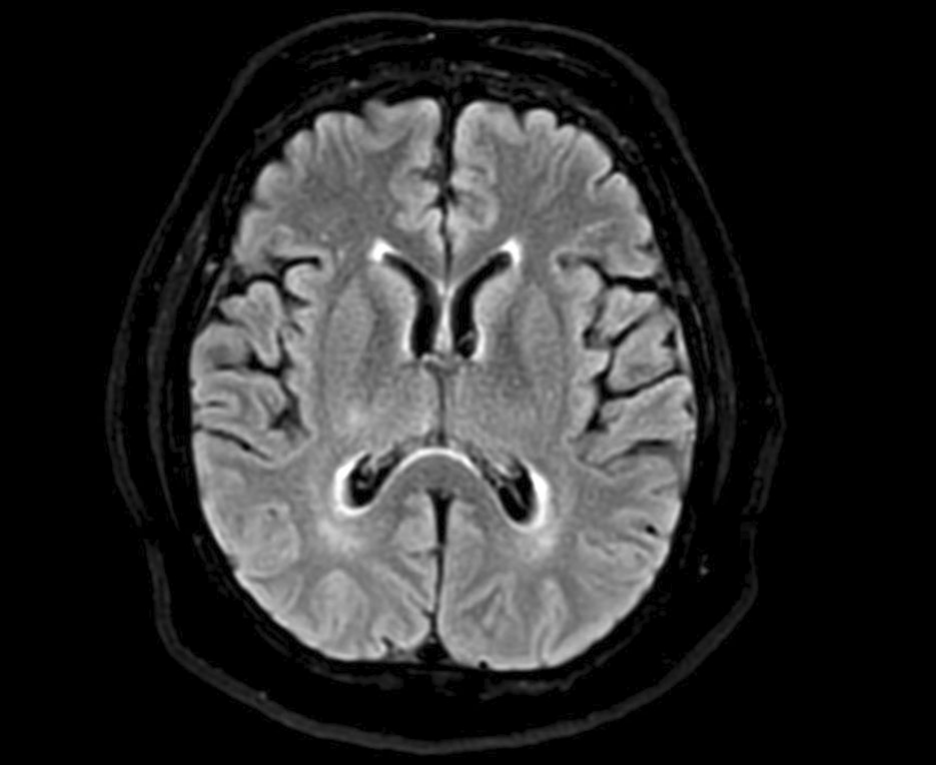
MRI brain w/wout contrast. Notable for chronic lateral right thalamic infarct, mild generalized volume loss. No acute intracranial abnormality. Ambra Imaging, Dignity Health Systems/St. Joseph Hospital Medical Center.
